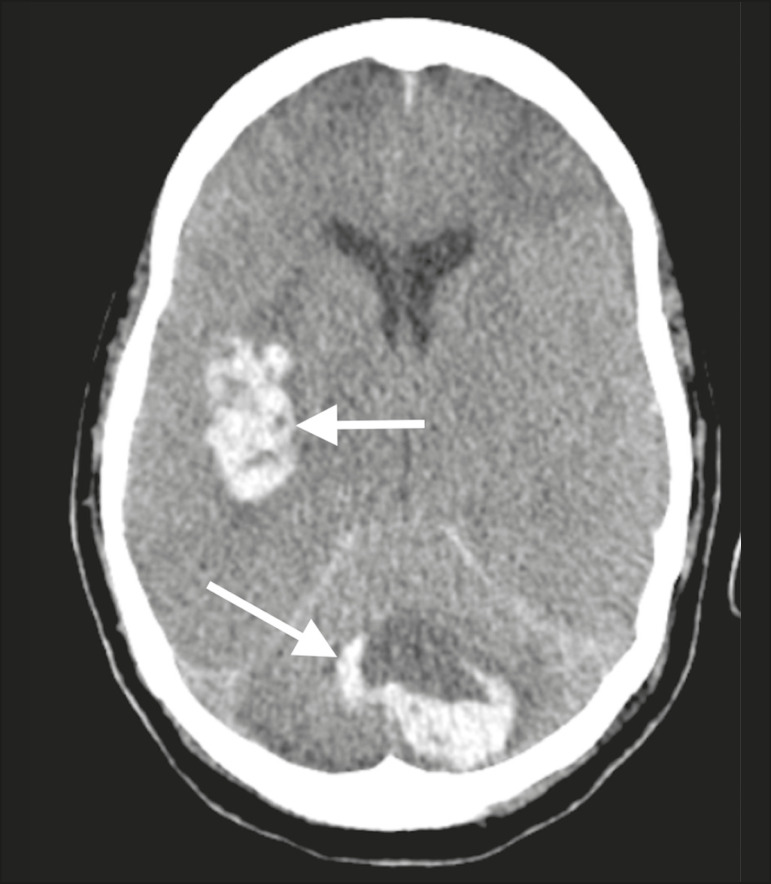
Case 6. Brain CT of a 61-year-old woman with COVID-19 showing supratentorial and infratentorial hematomas (arrows). The patient also had acute ischemic strokes in the left parieto-occipital and right parietal lobes, with hemorrhagic transformation (not shown).
